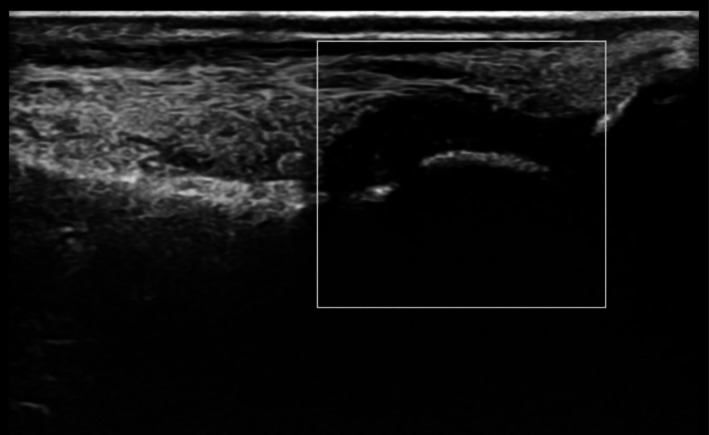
Power Doppler ultrasound of the second metatarsophalangeal (MTP) joints. Longitudinal view revealed significant synovial thickening and joint effusions, consistent with peripheral spondyloarthritis.
